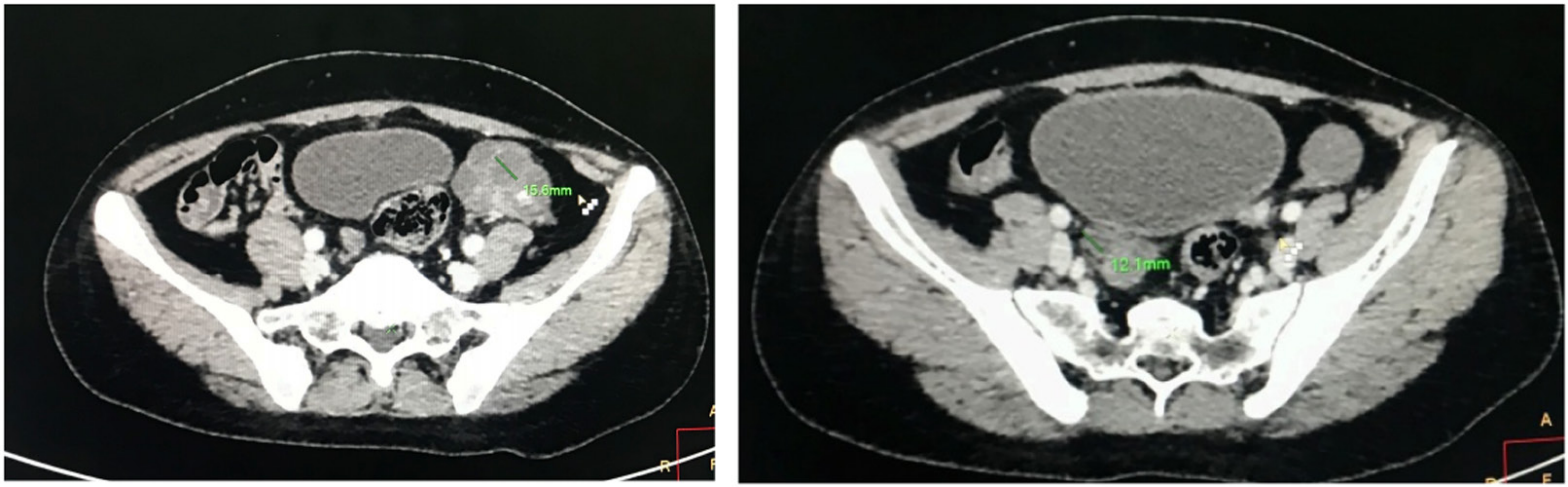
Axial CT image showing multiple non‐enhancing cystic lesions in bilateral ovaries, the largest measuring 15.6 mm and 12.1 mm in the left and right ovaries, respectively
